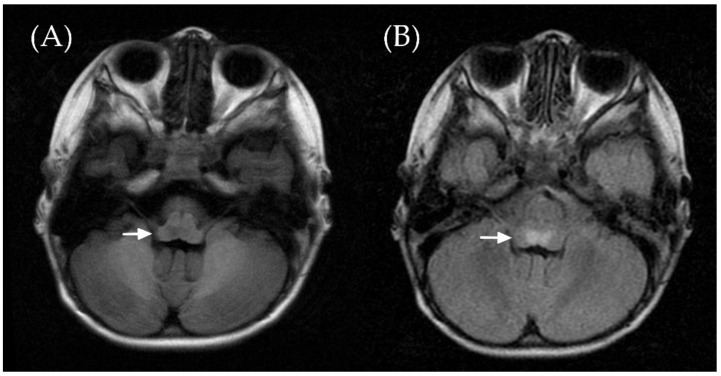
Brain magnetic resonance imaging showed symmetric lesions involving the dorsal pons and medulla oblongata, revealing brainstem encephalitis. These lesions (arrow) were hypointense in the T1-weighted FLAIR sequence panel (A) and hyperintense in the T2-weighted FLAIR images panel (B).
